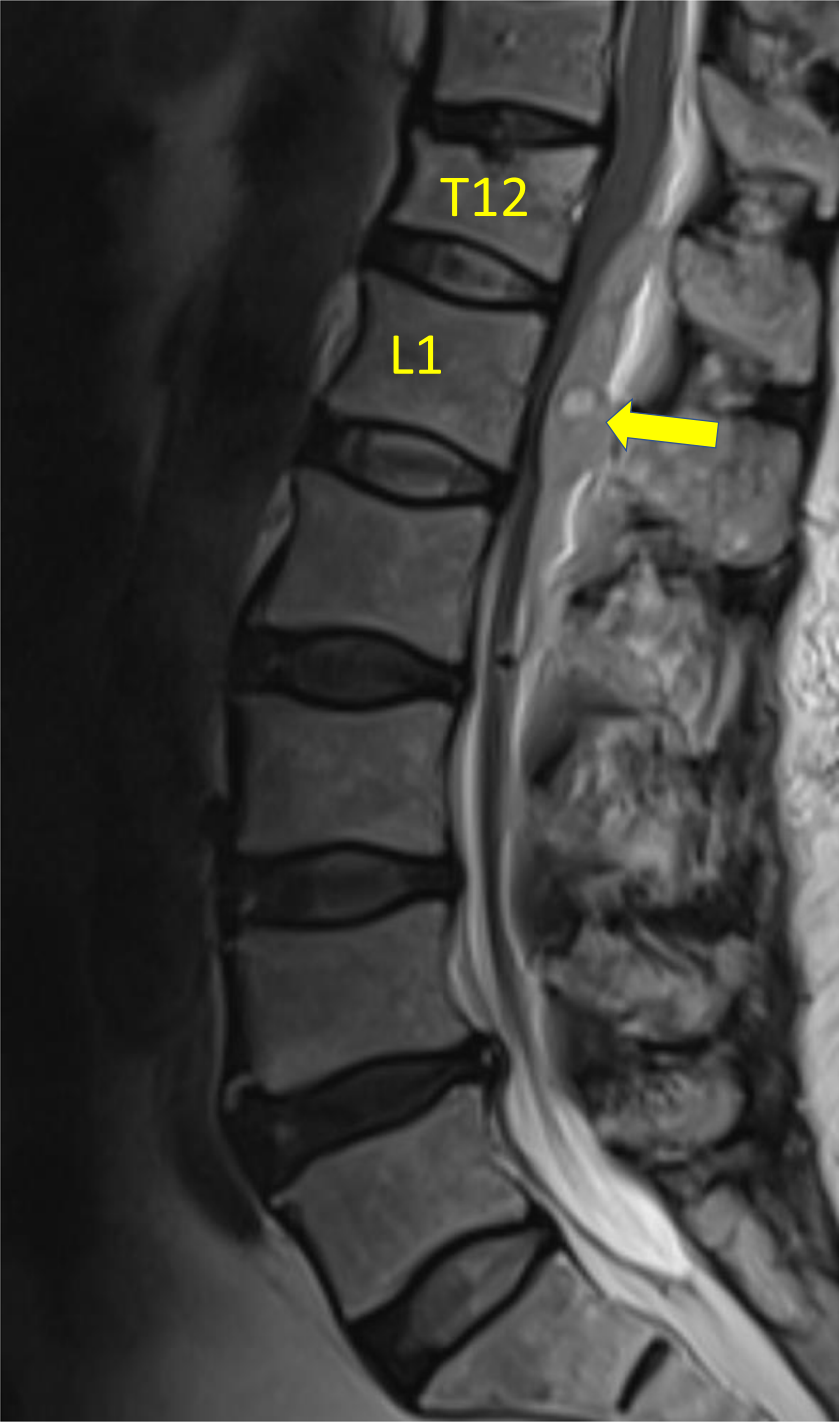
Lumbosacral magnetic resonance imaging without contrast, Sagittal view. T12: The 12th thoracic vertebrae, L1: The 1st lumbar vertebrae, arrow: an epidural hematoma extending from the levels of T12 to L2
